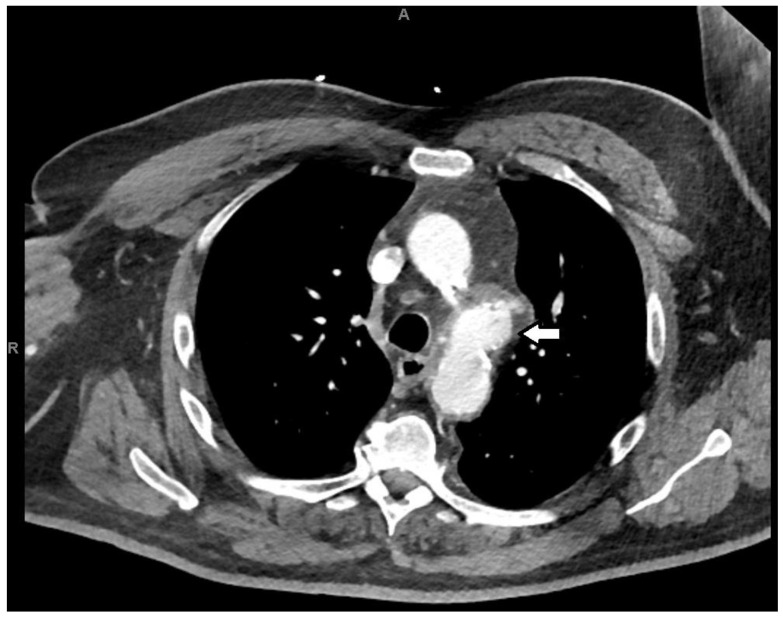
Cross-section of CT imaging with arrowhead highlighting the aneurysm of the distal aortic arch.
